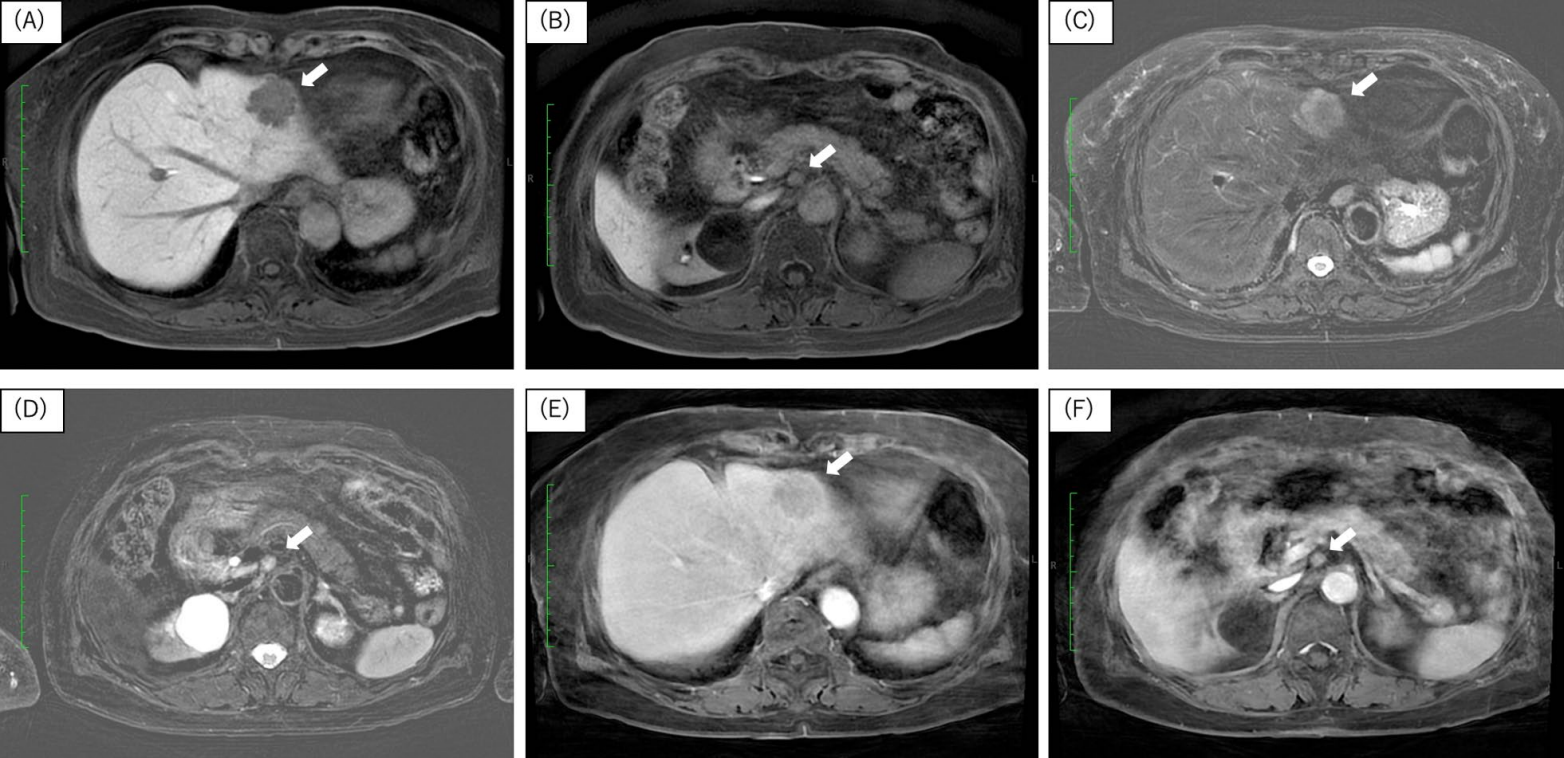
Preoperative MRI findings. A, B MRI (T1) showed the tumors as irregular margins and low signal lesions (white arrows). C, D MRI (T2) showed the liver tumor as heterogeneous high signal lesion and the retroperitoneal tumor as regular high signal lesion. E, F Contrast-enhanced MRI showed same findings as contrast-enhanced CT scan. They were suspected metastasis lesion of colon cancer
